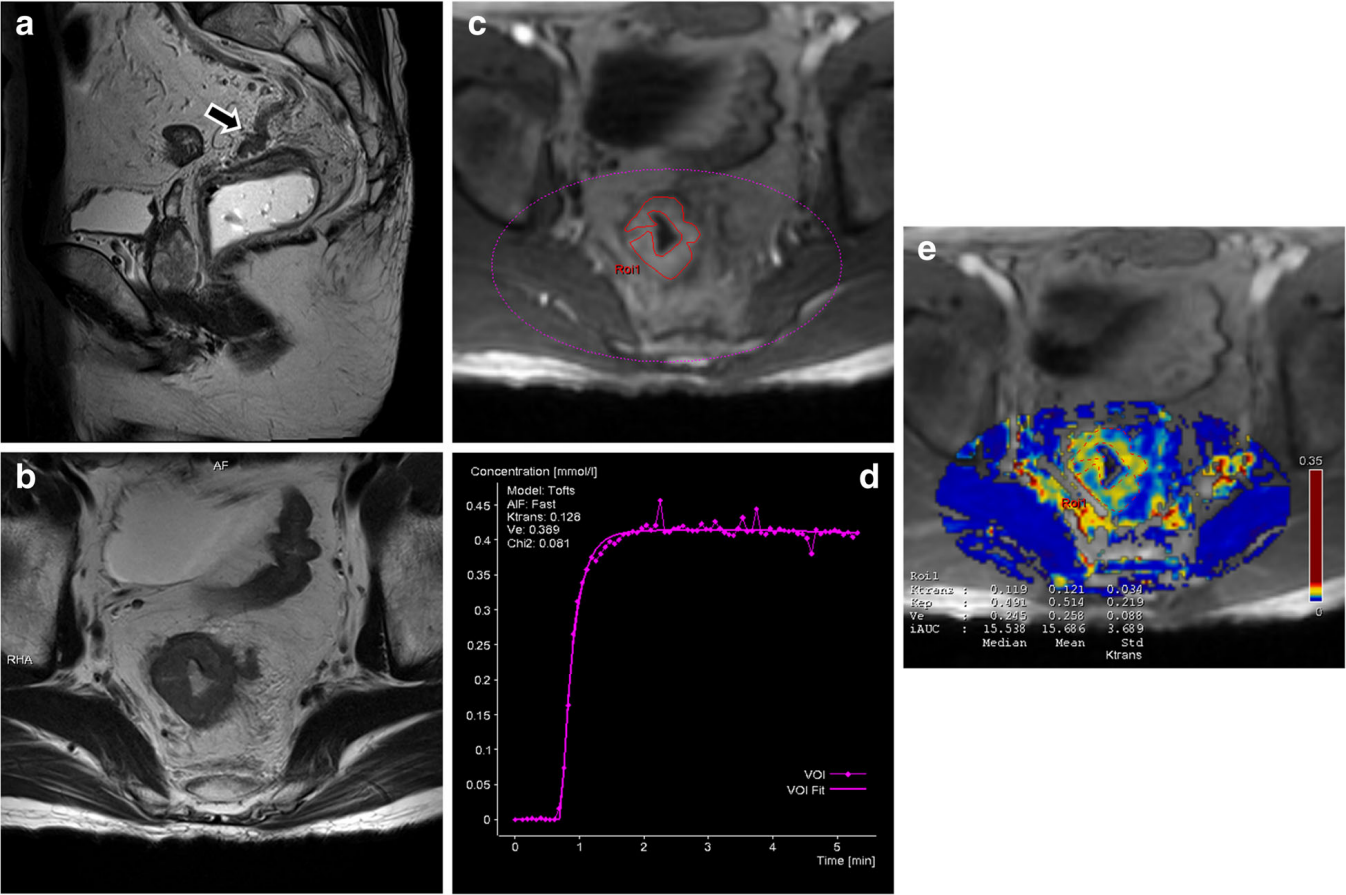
A patient with rectal adenocarcinoma (pT4N1, moderately differentiated). a Sagittal high-resolution T2WI depicted definitive tumour signal within a marked expanded and irregular extramural vessel (arrow), confirming mrEMVI-positive status. c A VOI (pink dashed line) was selected on DCE-MRI, and (d) the AIF with the smallest chi-square value was the selected. c An ROI (red continuous line) was drawn along the edge of the tumour guided by corresponding (b) oblique axial high-resolution T2WI. e The ROI was copied to *K*^*trans*^ map, and the mean *K*^*trans*^ (0.121 min^− 1^), *k*_*ep*_ (0.514 min^− 1^) and *v*_*e*_ (0.258) values were obtained
